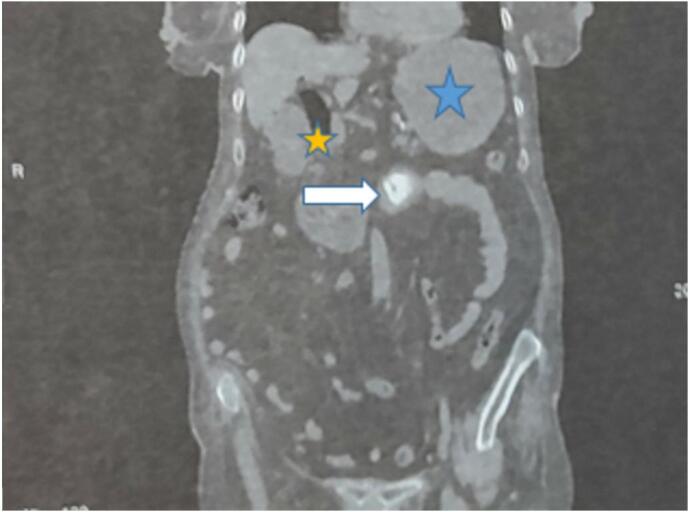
CT scan showing Rigler triad: pneumobilia/ gastric distention /large gallstone in dudenojujenum junction.
